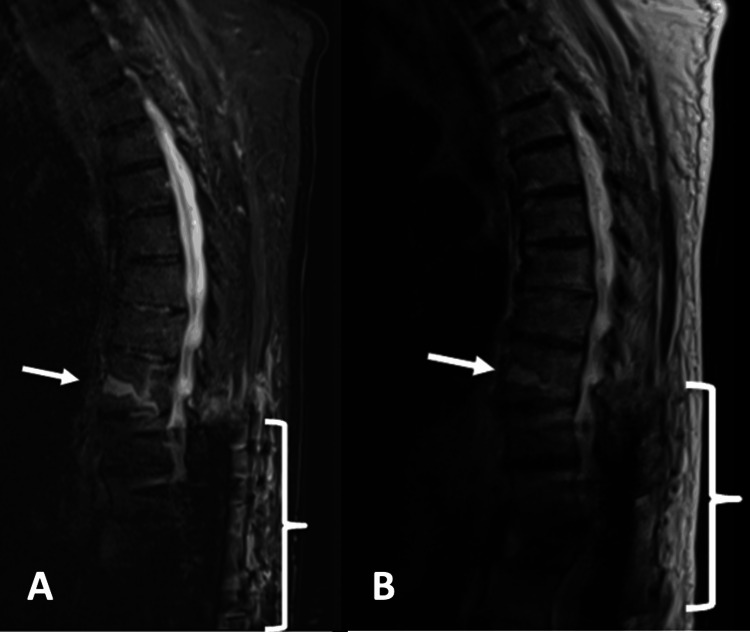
Postoperative T2W/STIR and T2W and MRI thoracic spine. Postoperative T2-weighted (T2W)/Short Tau Inversion Recovery (STIR) (A) and T2W (B) MRI thoracic spine of this 79-year-old man, acquired two weeks postoperatively, demonstrating a T9 vertebral body fracture with internal fluid material (white arrows), presumably hemorrhage, and T10-L2 fusion hardware (white bracket) surrounding the T12 pathologic compression deformity.
